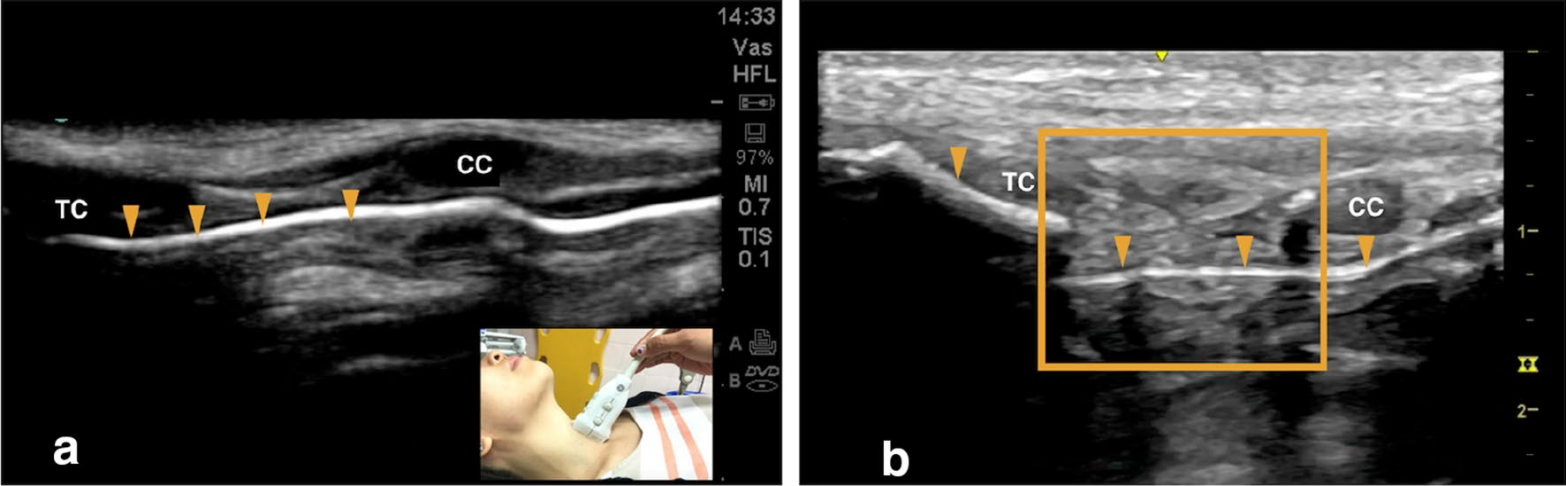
a Normal sonoanatomy in longitudinal scan showing continuous and intact air–mucosal interface (arrowhead) in relation to thyroid and cricoid cartilage. b Airway ultrasound image in longitudinal scan showing disruption of the air–mucosal interface (arrowhead) and formation of endolaryngeal hematoma and fracture cricoid cartilage (box). TC: thyroid cartilage; CC: cricoid cartilage
